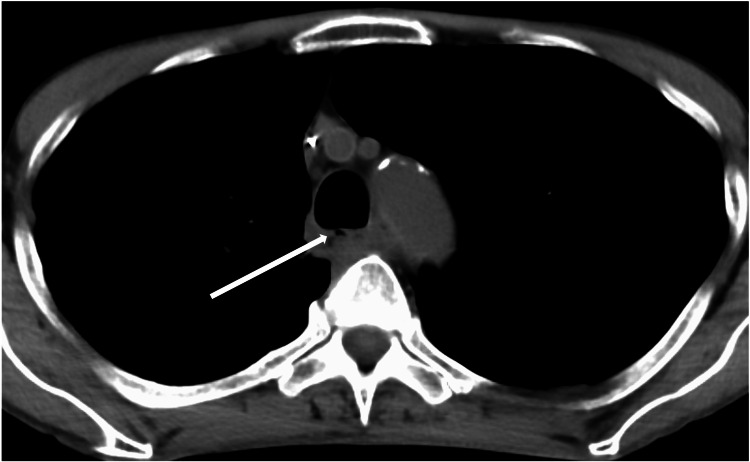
Chest computed tomography (CT) findings A small mass shadow is observed in the upper lobe of the right lung, and a fistula is observed between the right main bronchus and upper thoracic esophagus.
